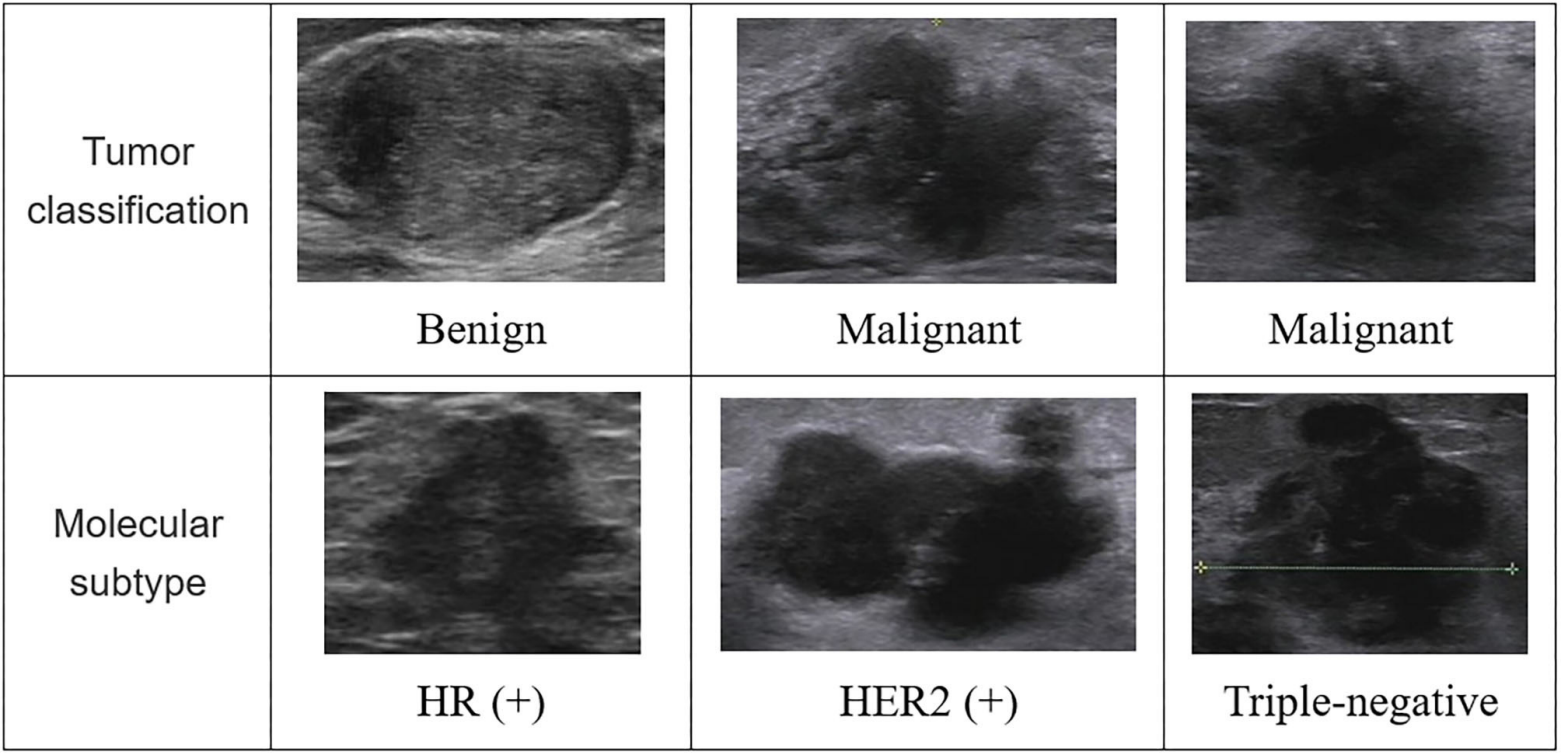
Some samples of breast ultrasound images from the datasets. In the first column, the samples were divided according to the diagnosis results of the tumor, which were benign and malignant; in the second column, the samples were classified according to molecular subtypes, which were divided into images of HR (+) subtype, HER2 (+) subtype, and triple-negative subtype. HR, hormone receptor; HER2, human epidermal growth factor receptor 2.
